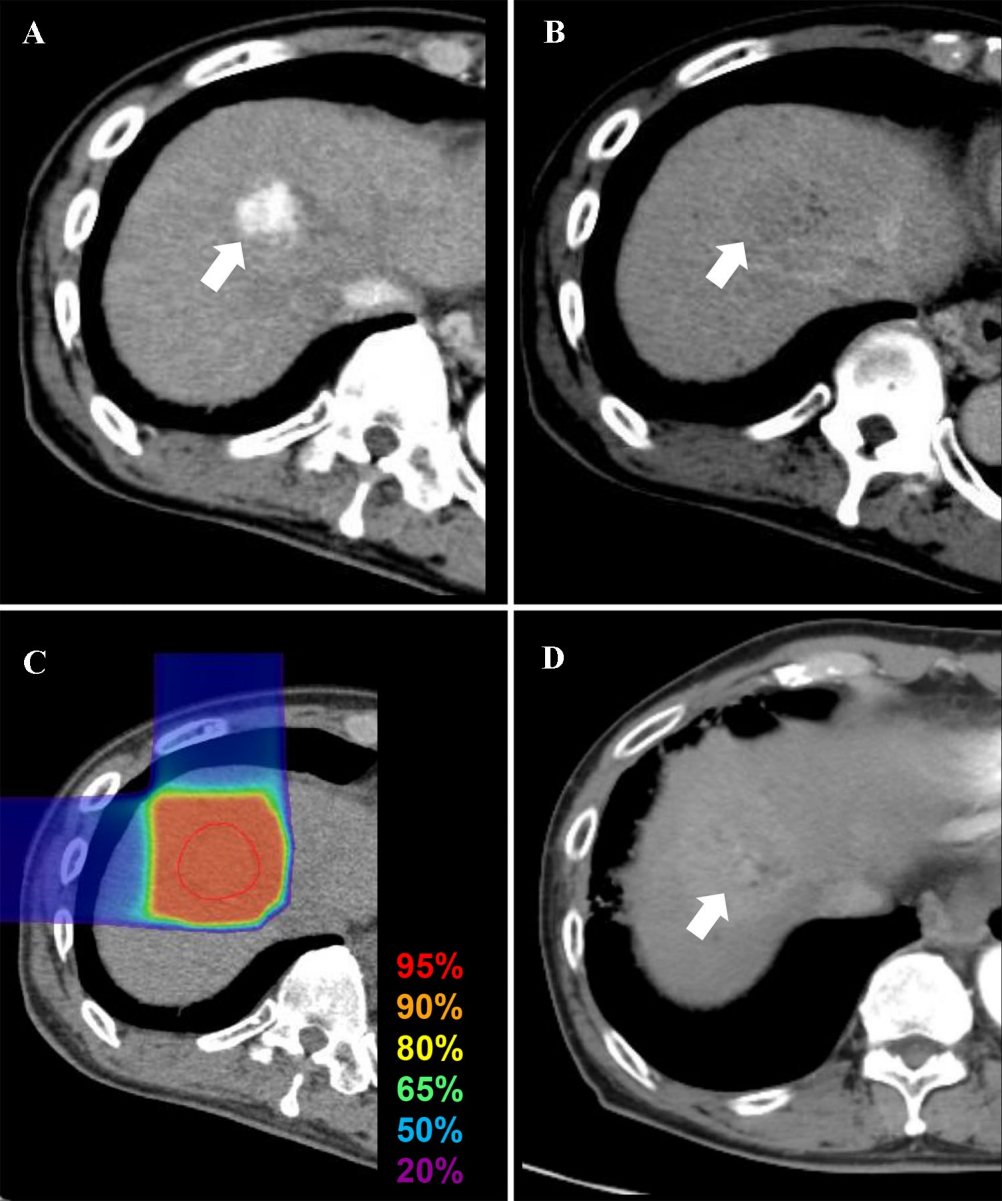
Contrast-enhanced computed tomography (CT) and dose distribution of carbon-ion radiotherapy (C-ion RT) for hepatocellular carcinoma (HCC). a Axial CT image taken in the arterial phase before C-ion RT. The white arrow shows HCC with arterial enhancement. b CT image taken in the delayed phase. The white arrow shows slight washout. c Dose distribution of C-ion RT. The area within the red outline is the gross tumor volume (GTV). Highlighted are the 95% (red), 90% (orange), 80% (yellow), 65% (green), 50% (blue), and 20% (purple) isodose curves (100% = 60.0 Gy [RBE]). d CT image taken in the arterial phase 6 months after primary C-ion RT, showing disappearance of the tumor
